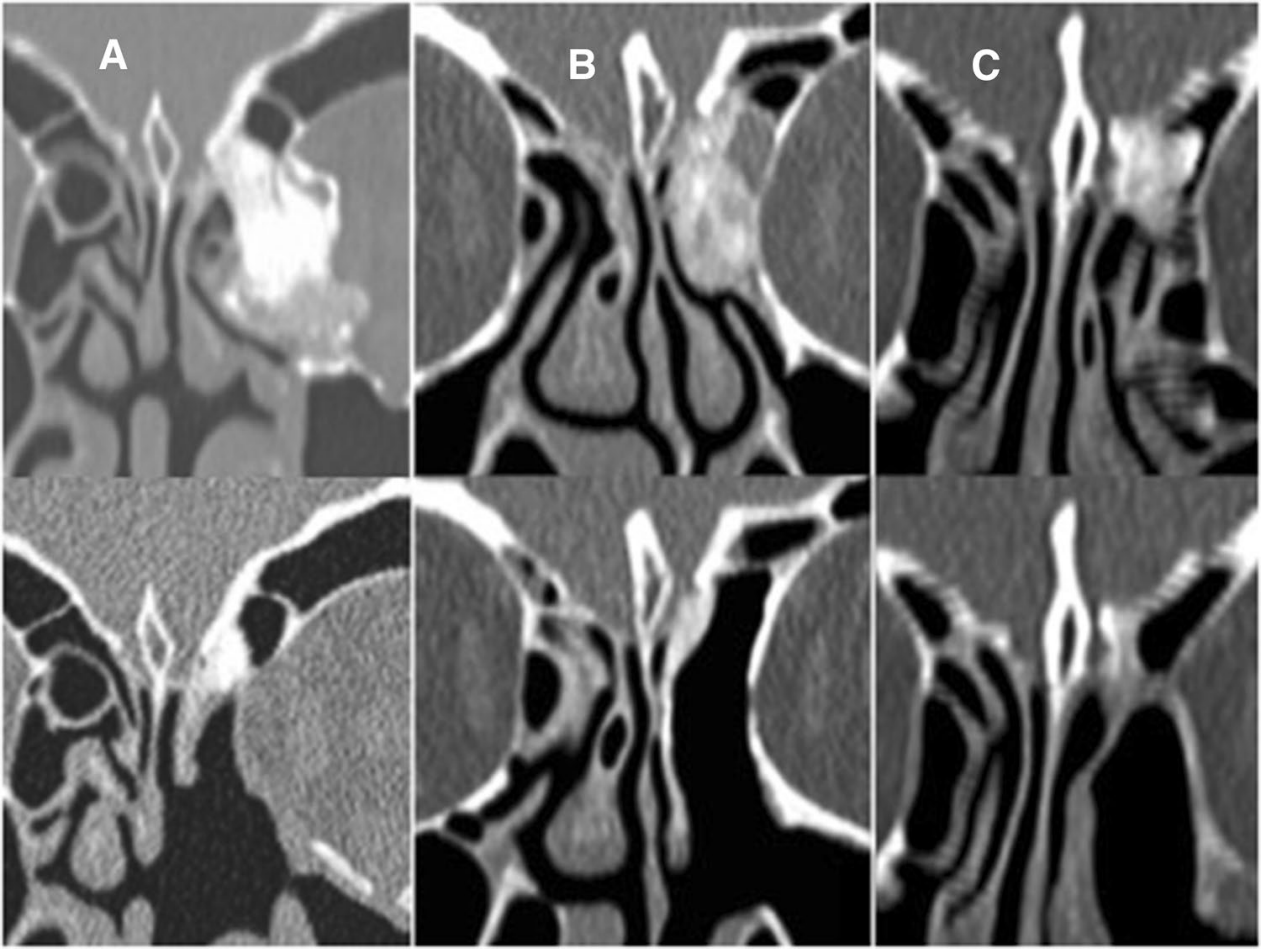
a–c CT of patients with osteomas with spongious component at the OG before and after surgery
